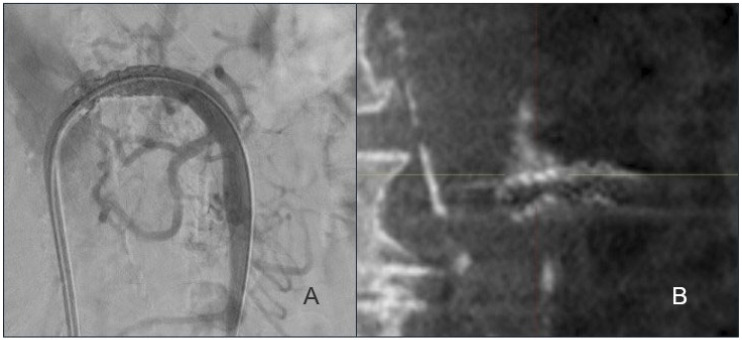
Completion imaging after SMA stent placement. Completion angiography (DSA) following stent placement (A). Stent placement visualized on CBCT (B).
